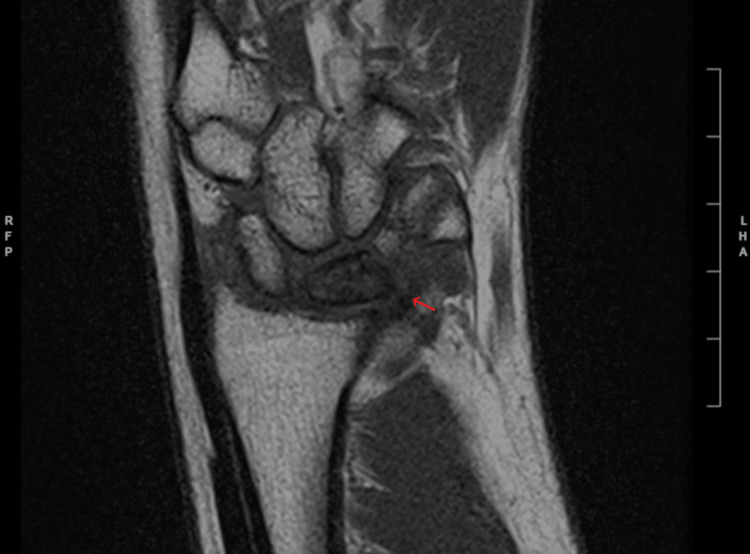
Post-treatment T1 coronal MRI The arrow corresponds to the diffuse low signal intensity of the lunate, which is similar to the previous MRI. Image # 10/19; spin echo (SE): 3001
